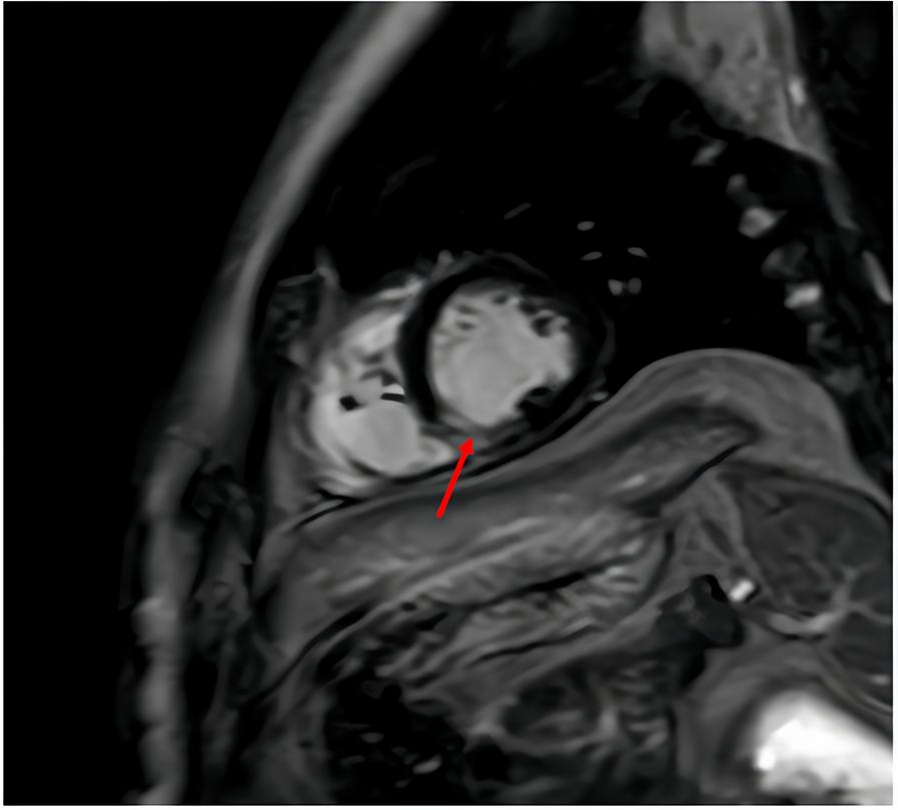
Cardiac magnetic resonance imaging (MRI) with contrast enhancement obtained during hospitalization. The arrow indicates transmural delayed enhancement in the mid-to-distal inferoseptal and mid-inferior segments of the left ventricle, which is indicative of myocardial infarction.
